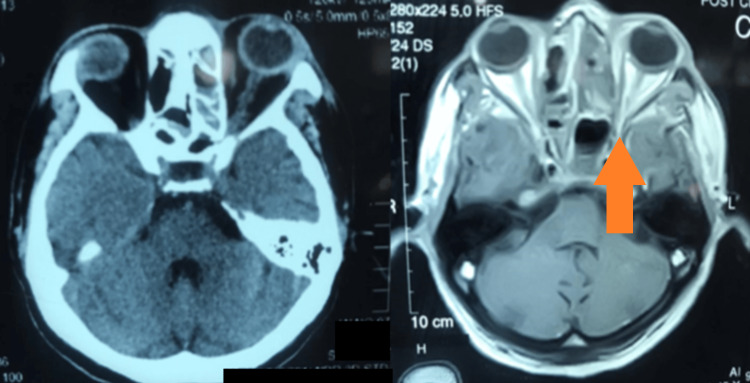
CT scan and MRI axial view of same patient showing double densities with loss of contrast enhancement on MRI T1WI Orange arrow: double densities CT, computed tomography; MRI, magnetic resonance imaging; T1WI, T1-weighted image
